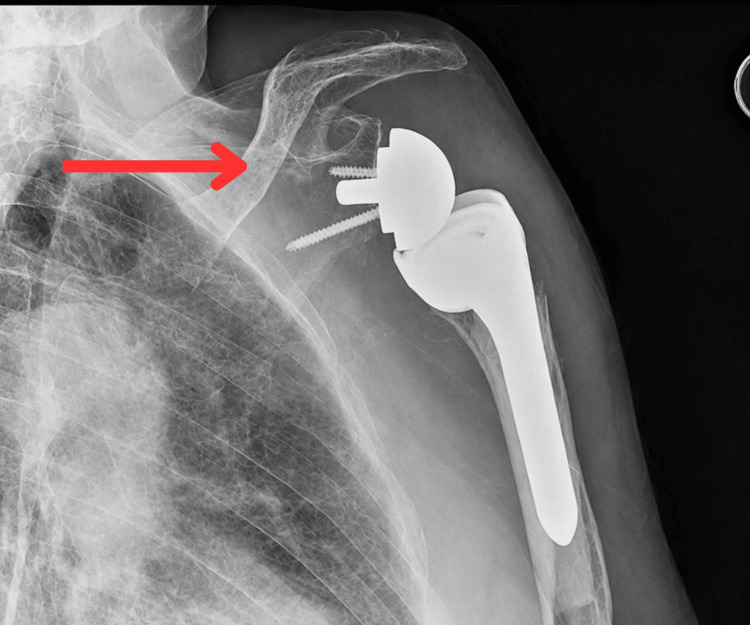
Immediate post-operative radiograph following the second open revision with glenosphere cup change
